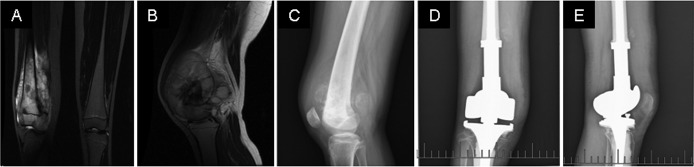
A patient who underwent knee arthroplasty (A and B) Preoperative MRI images show the tumor having crossed the physis. (C) A lateral X-ray image shows the tumor having crossed the physis. (D) An anteroposterior X-ray image shows the situation of the patient 6 months following knee joint and tumor resection, and reconstruction of the defect with metal prosthesis. (E) A lateral X-ray image shows the situation of the same patient 6 months following knee arthroplasty. MRI, magnetic resonance imaging.
